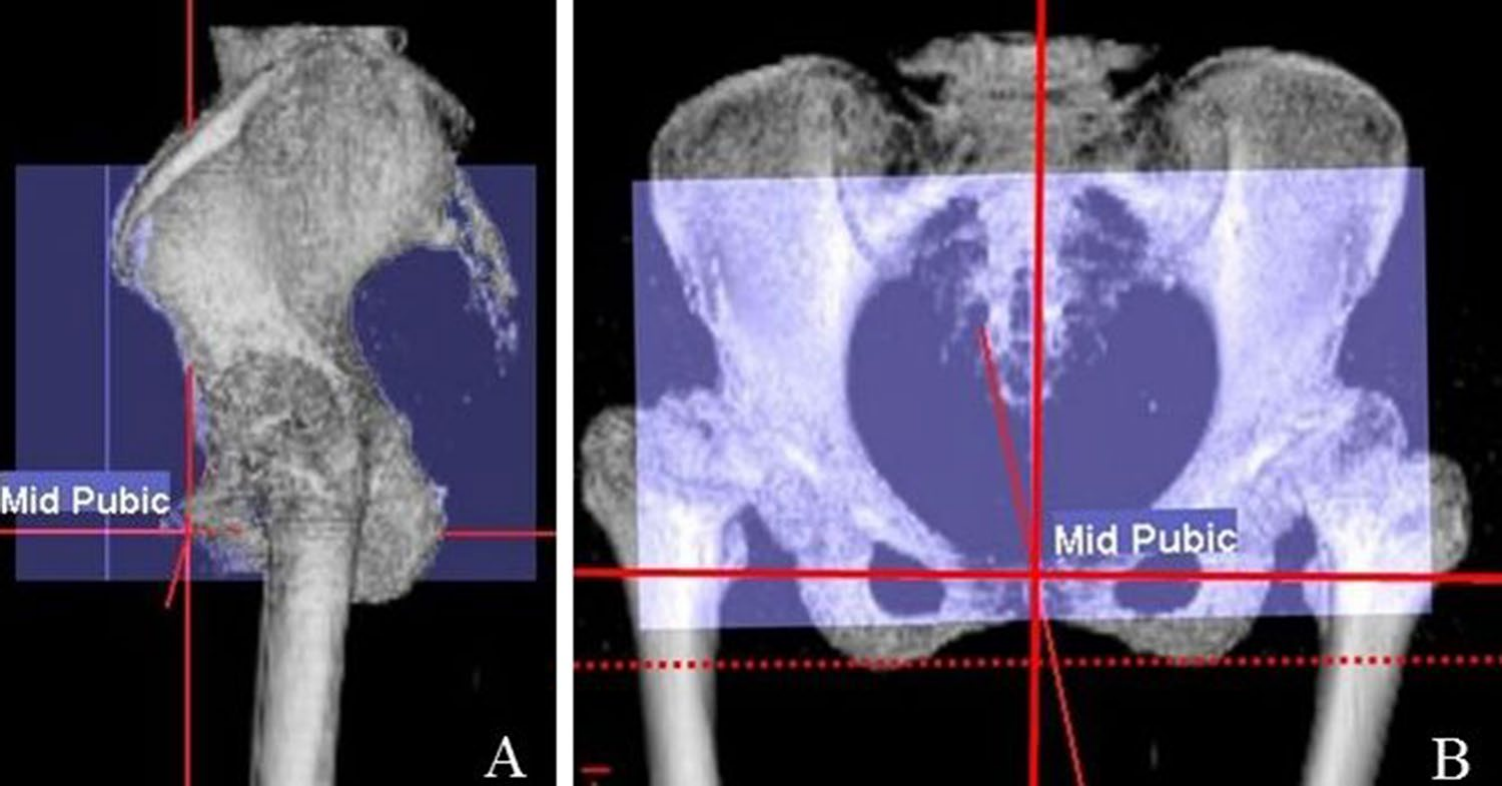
Patient positioning. a The pelvis was visualized in a functional plane in which the patients were placed in the supine position on the computed tomography (CT) scan table and the pelvis is axially rotated until the bilateral anterior superior iliac spines touch the same horizontal plane. b The pelvis was visualized in a functional plane, such that the lower edges of the bilateral ischia were horizontally aligned
